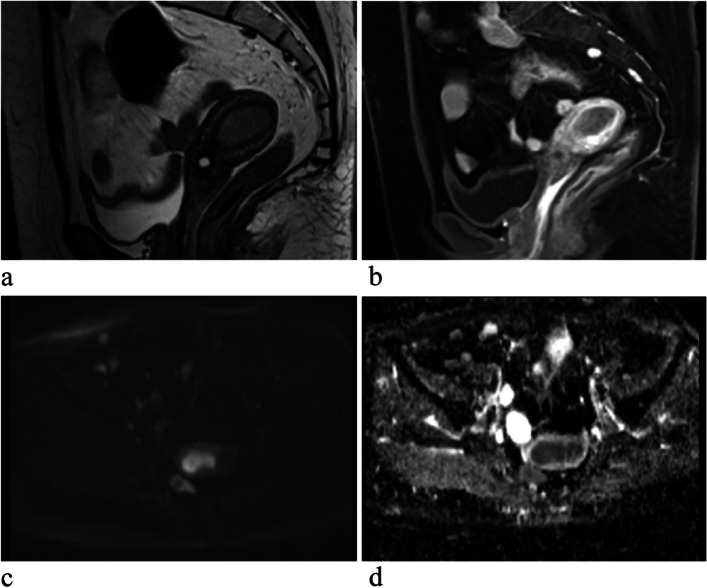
A patient with endometrioid adenocarcinoma (EAC). a Sagittal T2WI shows a homogeneous and slightly higher signal tumor. b Sagittal contrast enhanced T1WI, there is homogeneous mild enhancement in the mass lower than the myometrium. c DWI shows a obvious high signal intensity tumor. d ADC displays homogeneous low signal intensity in the tumor
